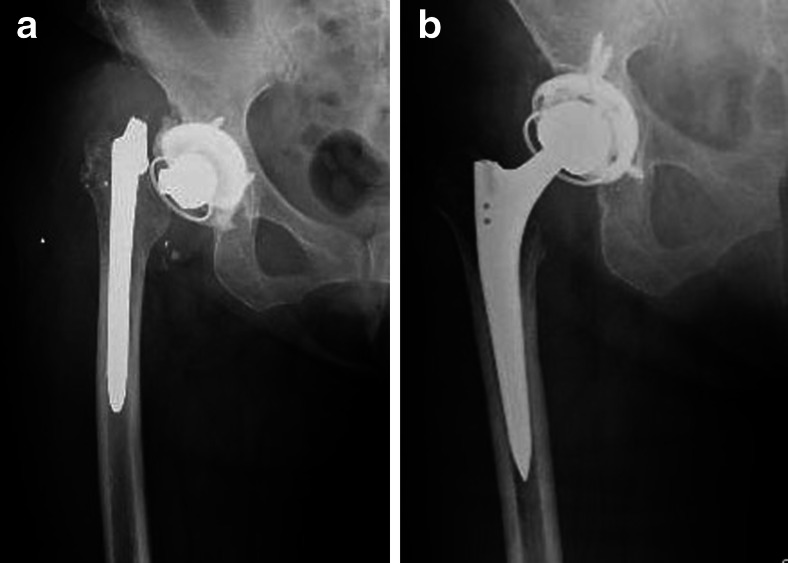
Radiograph of a 70-year-old female showing a femoral stem fracture after multiple dislocations of a THA (a) and successful reimplantation with a cementless stem was performed (b)
